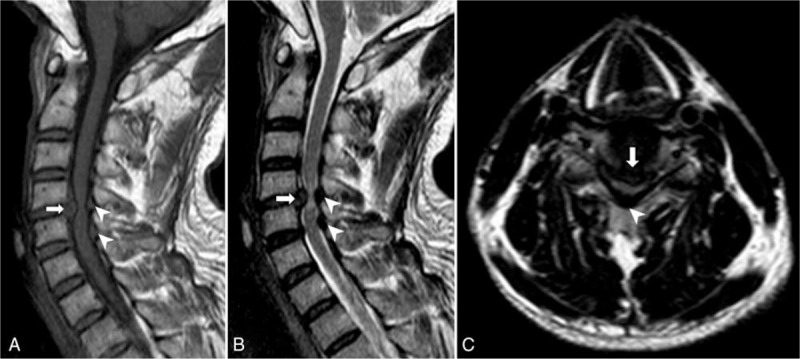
MR imaging showed C4/5 disc herniation (arrow) and thickened ligamentum flavum at C4–5 and C5–6 levels (arrow head), on T1W (A), T2W (B), and axial (C) images. MR = magnetic resonance.
